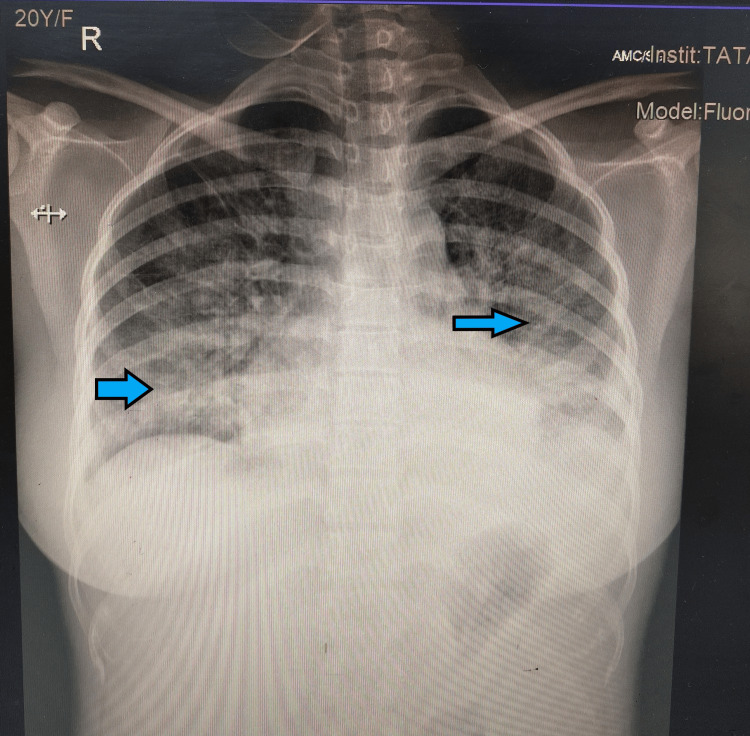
Anterior-posterior chest X-ray of the patient showing bilateral diffuse ground-glass opacities.
